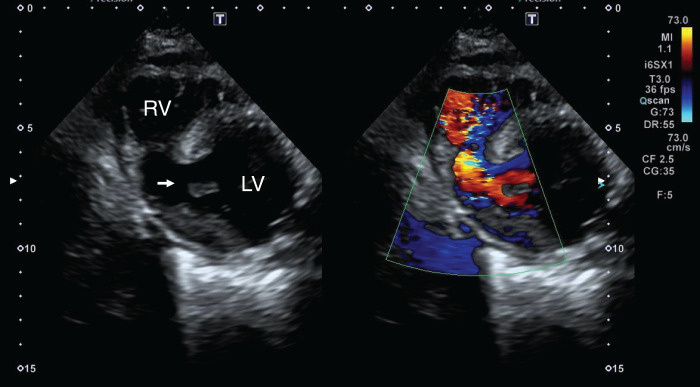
Echocardiography trans-thoracic echocardiography revealed decreased right ventricular contractility and a left-to-right shunt through a posterior interventricular septal rupture (arrow). LV, left ventricle; RV, right ventricle
